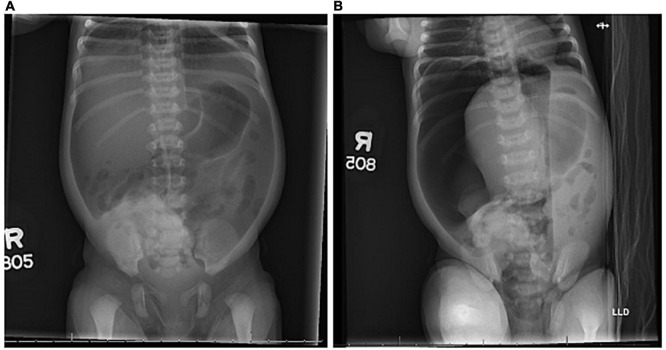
Radiographs. Supine (A) and left lateral decubitus (B) radiographs demonstrated pneumoperitoneum on day of life 3.
